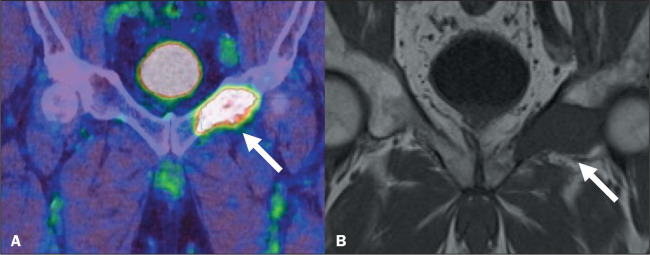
^68^Ga-PSMA PET/CT for prostate cancer staging in a
